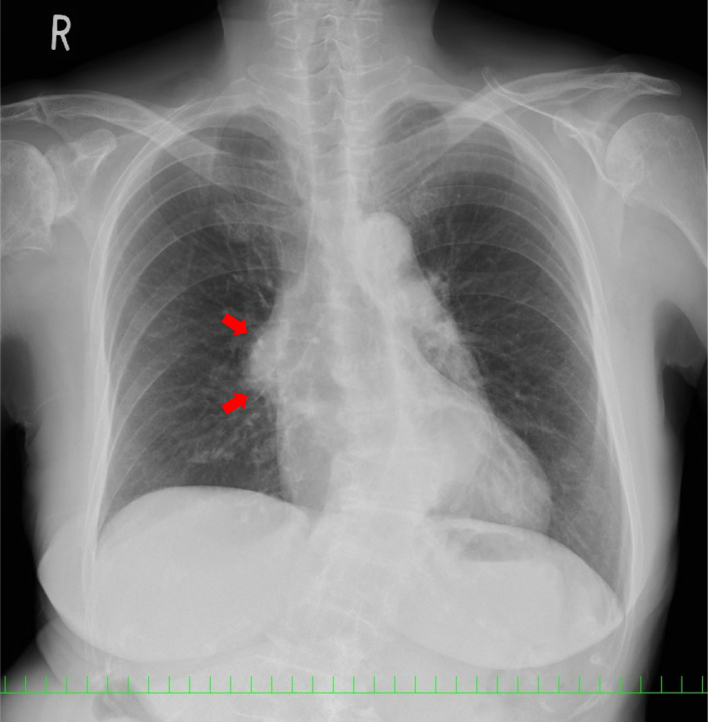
Chest radiograph showing a mass (red arrows) in the right hilum. (For interpretation of the references to colour in this figure legend, the reader is referred to the web version of this article.)
